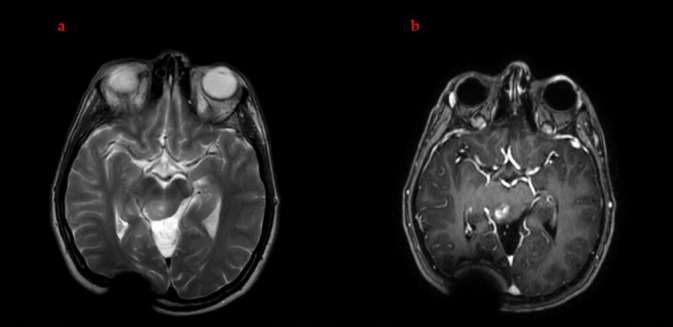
Seventh-month follow-up MRI, demonstrating significant radiological response following initiation of targeted therapy. (a) Axial T2-weighted image showing marked regression in the overall tumor dimensions. (b) Axial post-contrast T1-weighted image demonstrating nearly a 60% reduction in the enhancing component compared with earlier imaging.
